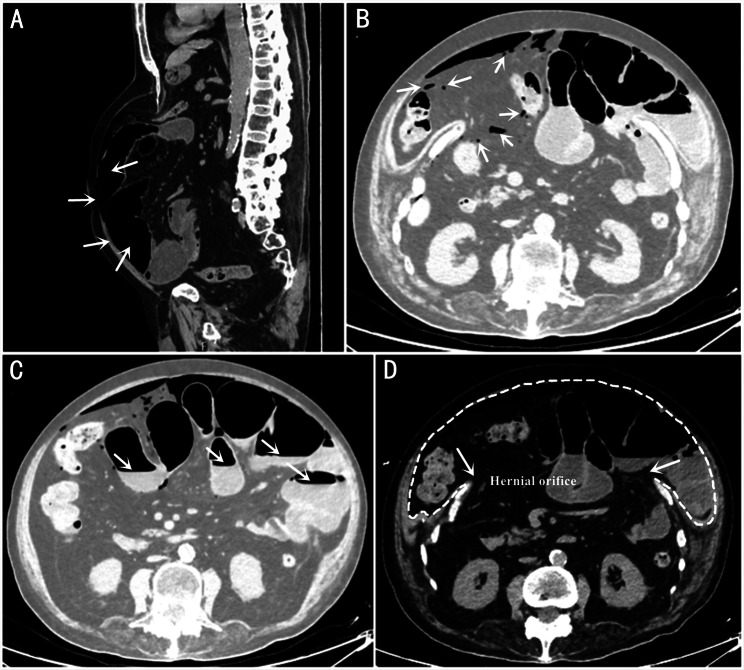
Imaging findings on contrast-enhanced CT. (A) the sagittal position showed dilated intestinal lumen, extensive gas within the intestinal lumen, and an abdominal wall fascial defect in the upper quadrant, (B) large amount of free gas visible in the abdominal cavity, (C) multiple air-fluid levels in the small bowel, (D) CT of the upper abdomen (axial view) showed an abdominal wall fascial defect, approximately 15 cm in the greatest diameter, and the small intestine was herniated into the subcutaneous layer to form a giant abdominal wall hernia, white arrowheads indicated the defect in the abdominal wall musculature and fascia (hernial orifice), the contour of the hernia sac was outlined by a white dashed line
